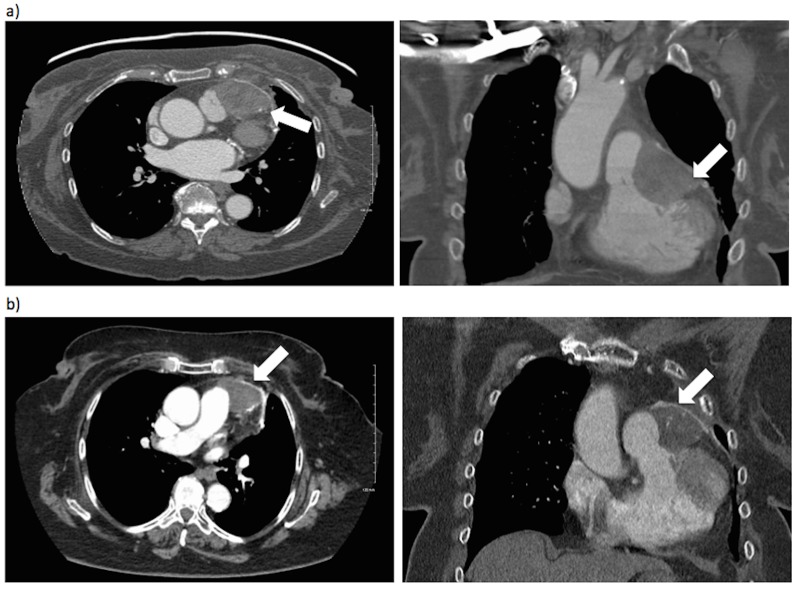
Axial and coronal computed tomography scans of the lesion (a) Pre-salvage radiotherapy, June 2012; (b) Post-salvage radiotherapy in follow-up, June 2015.
